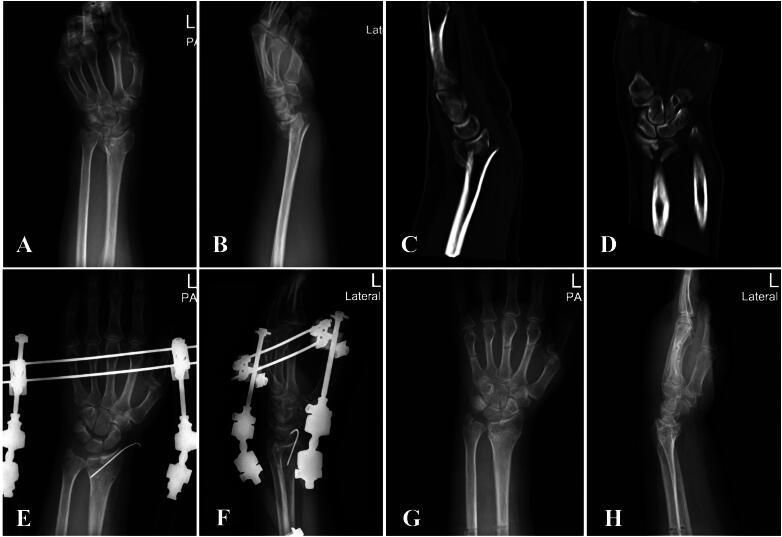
Radiographic evaluation of Case 1 treated with BEF. (A, B) Preoperative anteroposterior and lateral radiographs of a 58-year-old female patient with an AO type C2 DRF. (C, D) Preoperative coronal and sagittal CT images. (E, F) Postoperative anteroposterior and lateral radiographs showing successful fracture reduction and fixation with BEF. (G, H) Anteroposterior and lateral radiographs at 6 weeks postoperatively, showing maintained fracture alignment and early callus formation at the time of BEF removal. BEF: bi-frame external fixation; DRF: distal radius fracture.
